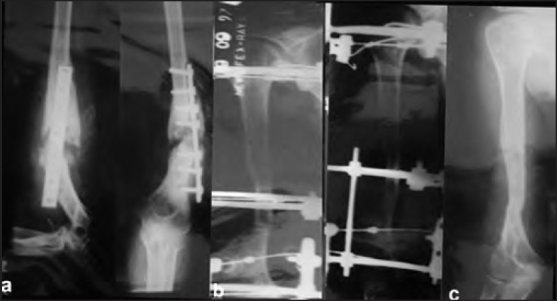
(a) Lateral and anteroposterior radiograph showing 10 month old post surgical infected nonunion (b) radiograph (lateral and anteroposterior view) showing ring fixator *in situ* (c) radiograph (anteroposterior view) showing radiological union at 26 weeks
